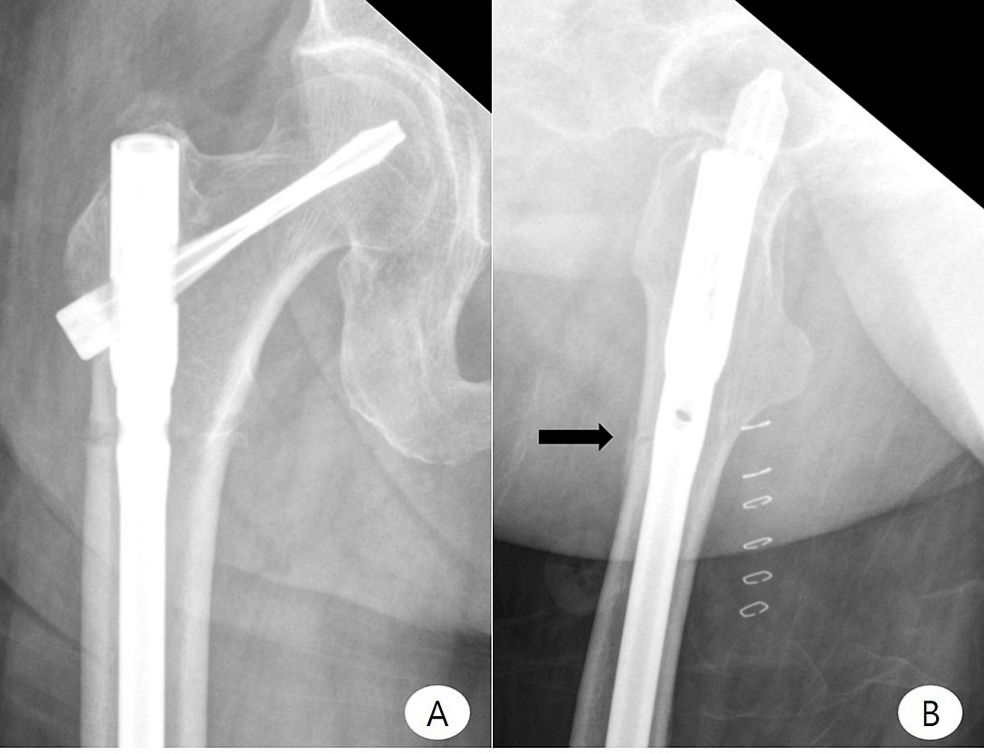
Postoperative imaging - case 1 Postoperative anteroposterior (A) and lateral (B) radiographs. Black arrow indicates cortical thickening and incomplete fracture line at the anterior femoral cortex. The images show complete healing of the previous incomplete fracture at the subtrochanteric region
